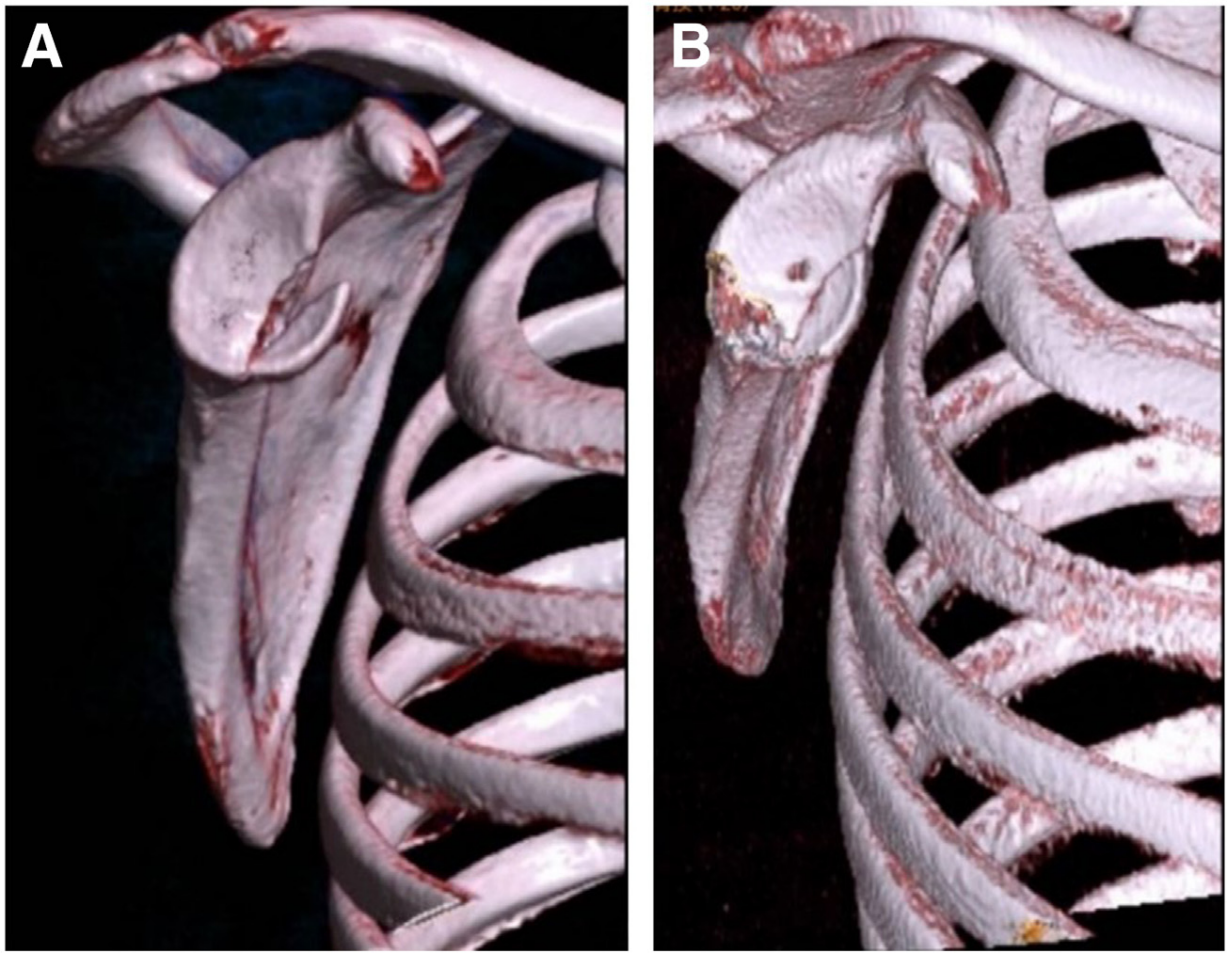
Preoperative and postoperative images of the bony Bankart lesion. (A) Preoperative images of the bony Bankart lesion on 3D CT reconstruction of the “en face view.” (B) Postoperative images of the bony Bankart lesion on 3D CT reconstruction of the “en face view,” showing anatomical reduction and stable fixation of the fragment. (3D, 3-dimensional; CT, computed tomography.)
